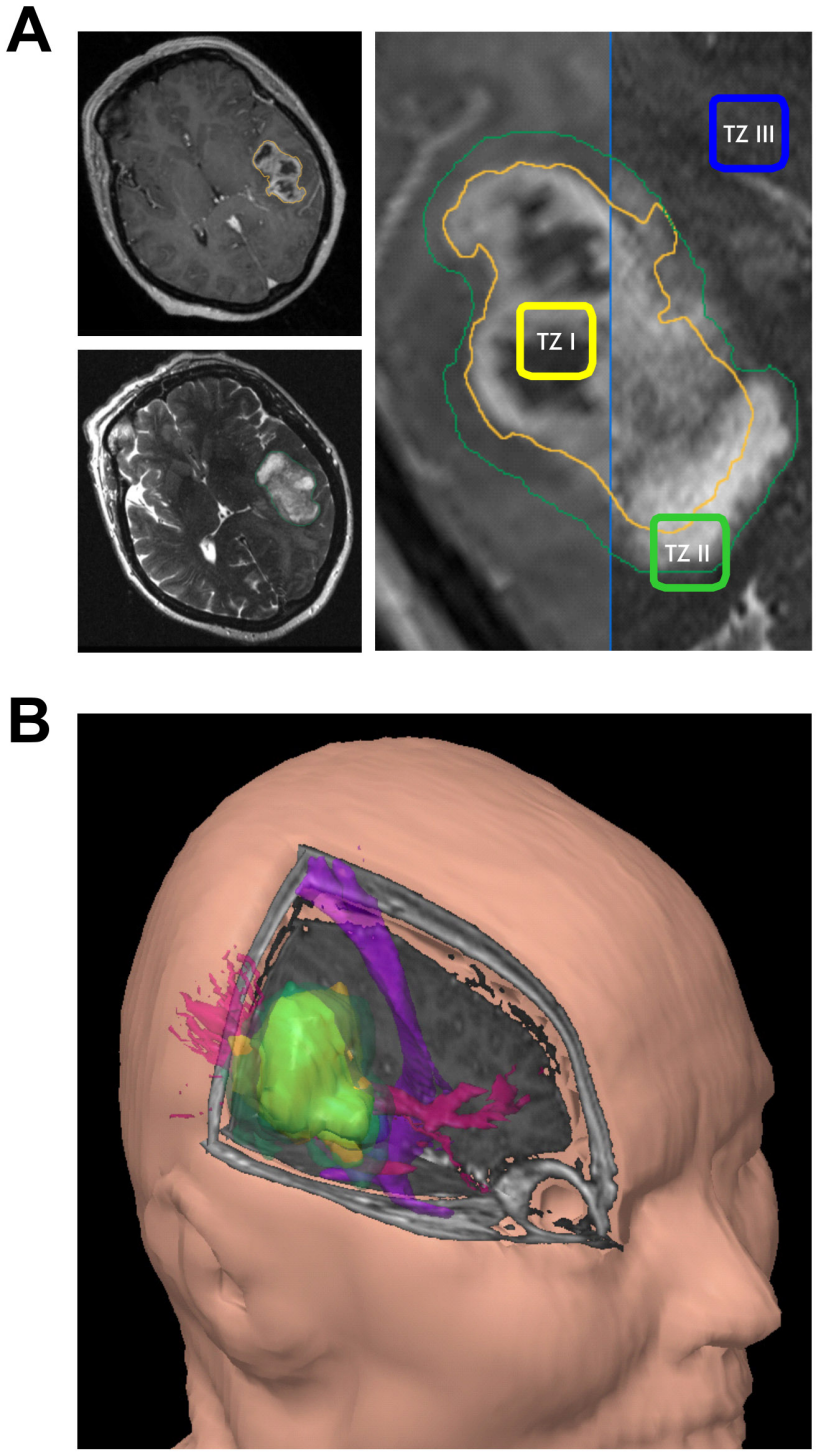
Visualization of differential tumor zones with respect to functionally eloquent areas of the brain. (A), T1 and T2 weighted MR images of a right temporo-dorsal GBM, fused in order to delineate the various tumor zones (TZ): TZ I – corresponding to contrast agent enhancing areas, TZ II – corresponding to the perifocal edema, TZ III – corresponding to apparently healthy brain parenchyma. (B), three dimensional representation of the same tumor in relation to anatomically relevant fiber tracts (pink: optic radiation, purple: pyramidal tract).
